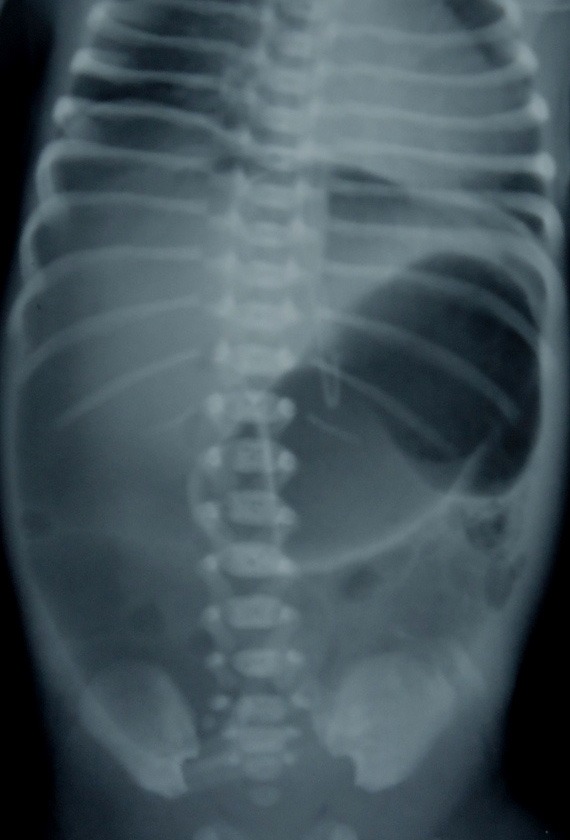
Figure 1: Plain X-ray abdomen showing pneumoperitoneum with loculated air pockets and distended stomach.
